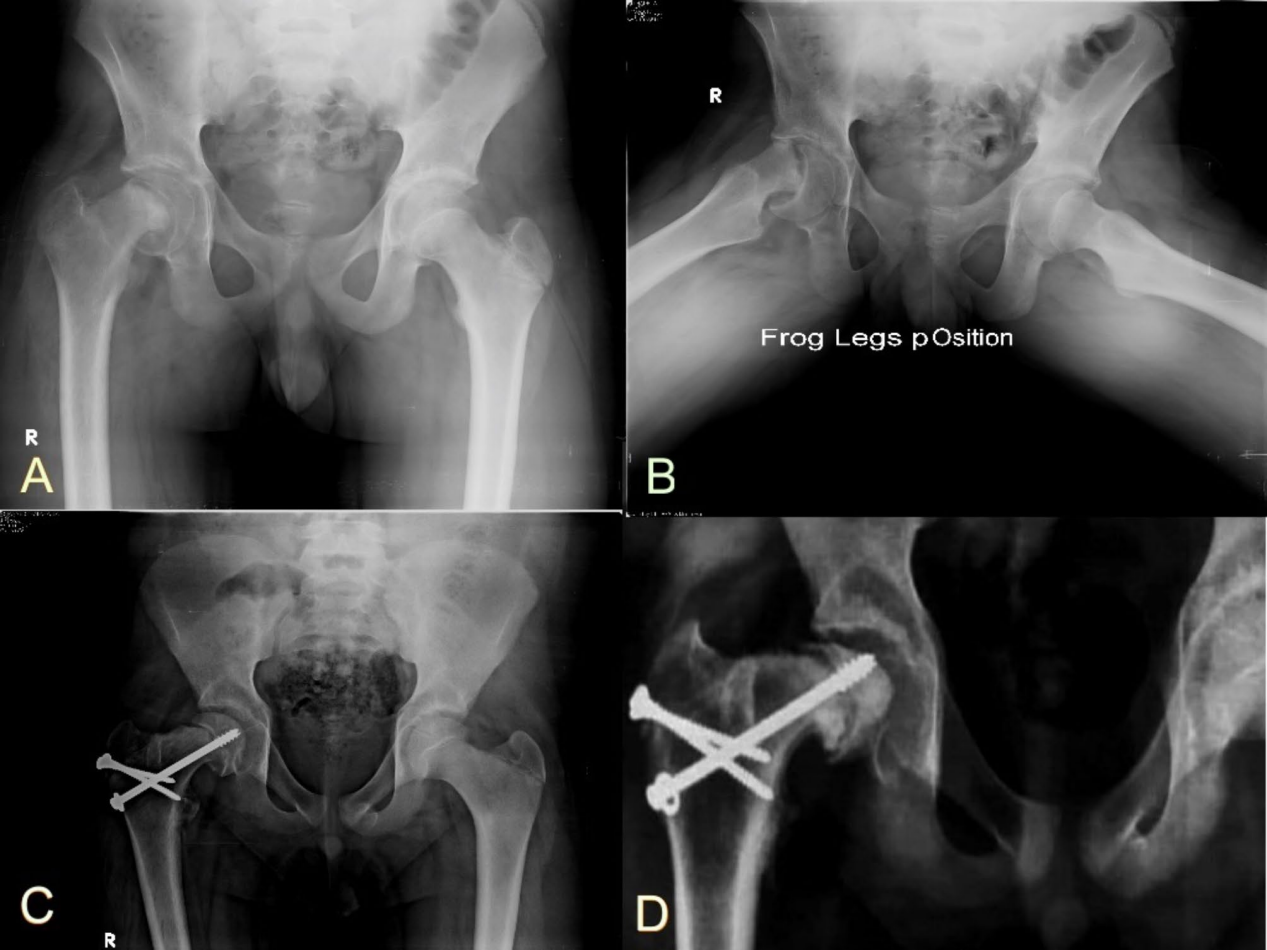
A) and B): Preoperative radiographs of a 12-year-old male patient who presented with acute on top of chronic SCFE. C) An immediate postoperative x-ray showed a varus reduction of the physis. D) A follow-up x-ray at ten months showed the development of AVN
